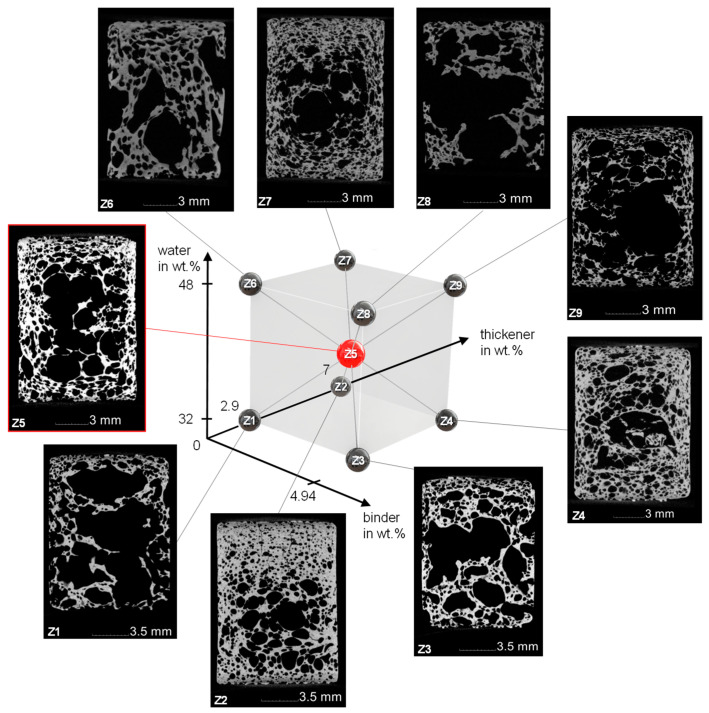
Structure of the DoE with corresponding CT images of the sintered Freeze Foams.
